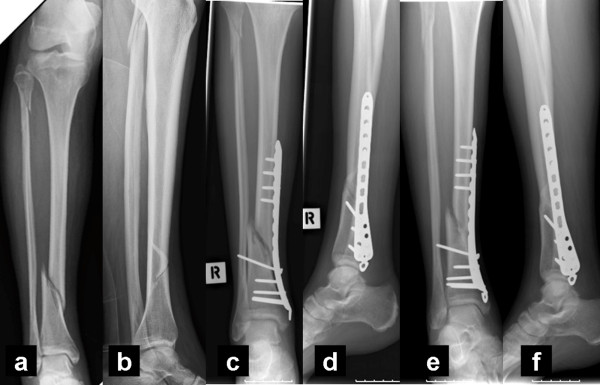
Anteroposterior (a) and lateral radiographs (b) of an open Grade I distal tibial fracture. Six months after surgery, anteroposterior ( c) and lateral tibial radiographs ( d) showed slow progression of healing. Seven months after PEMF introduction, anteroposterior ( e) and lateral tibial radiographs ( f) showed bridging callus in 3 out of 4 cortexes (medial, anterior and posterior).
